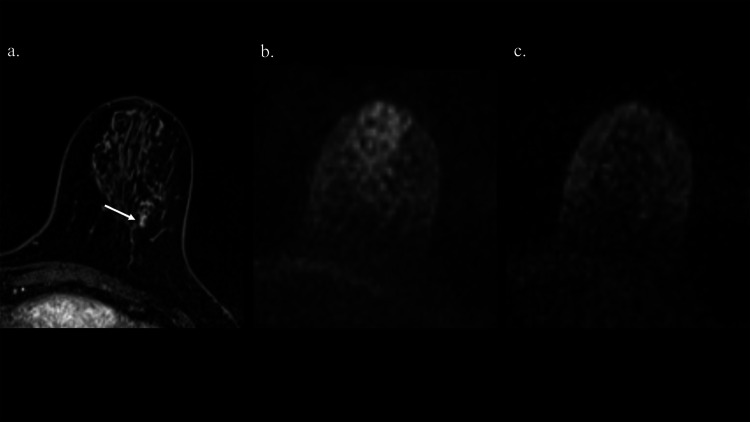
A 65-year-old woman underwent preoperative MRI for evaluation of ductal carcinoma in situ in the right breast. On early-phase DCE-MRI, an 8.2-mm non-mass enhancement (NME) was detected in the left breast (contralateral to the known breast cancer) (a, arrow) and assessed as BI-RADS category 4. On both DWI (b, DWI_800_ and c, DWI_1500_), the lesion was isointense to the surrounding fibroglandular tissue and not visually distinguishable. Both readers assigned a DWI visibility score of 0 on both DWI. On MRI-targeted ultrasonography, the lesion was depicted as an approximately 5-mm hypoechoic area and was diagnosed as invasive lobular carcinoma by ultrasound-guided vacuum-assisted biopsy (DWI false-negative)
